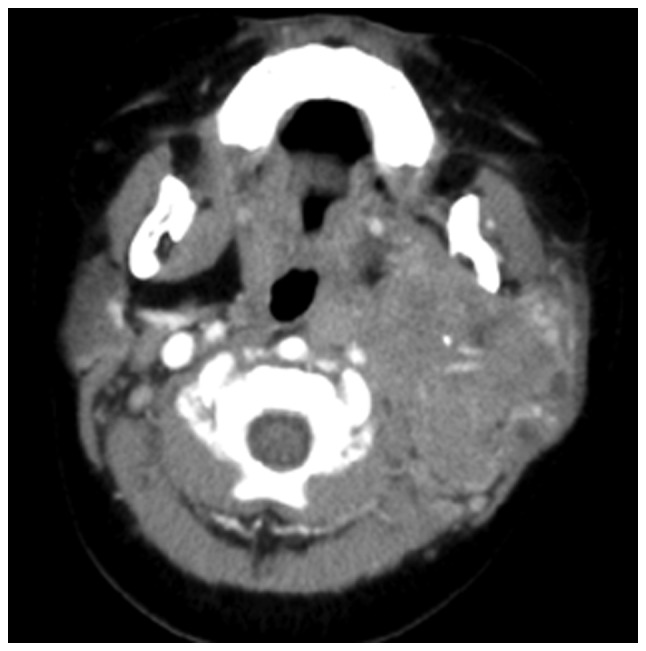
Axial computed tomography (CT) scan showing a large heterogenous mass in the left parotid region crossing the carotid arteries, without the underlying bone.
